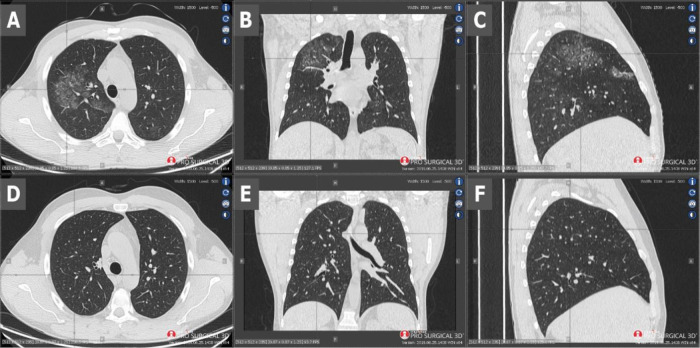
Scans A-C - CT performed on admission - scans show diffuse ground-glass opacities and septal interlobular thickening in the right lung, as well as paving-stone findings which could represent alveolar haemorrhage. Scans D-F - CT performed 2 months later - visible radiological regression.
